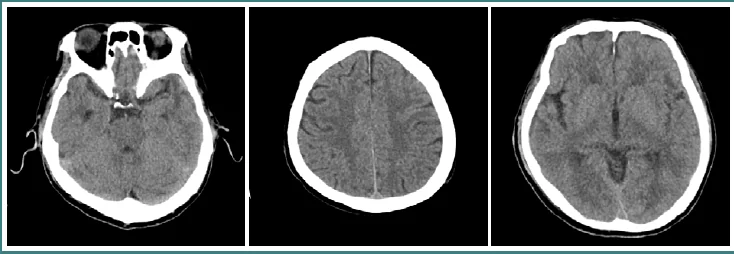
Follow-up brain CT (on day 28 after injury), obtained during rehabilitation, demonstrated progressive resorption. Brain CT showed decreased attenuation of hemorrhagic contusions in the left frontal and temporal lobes.
